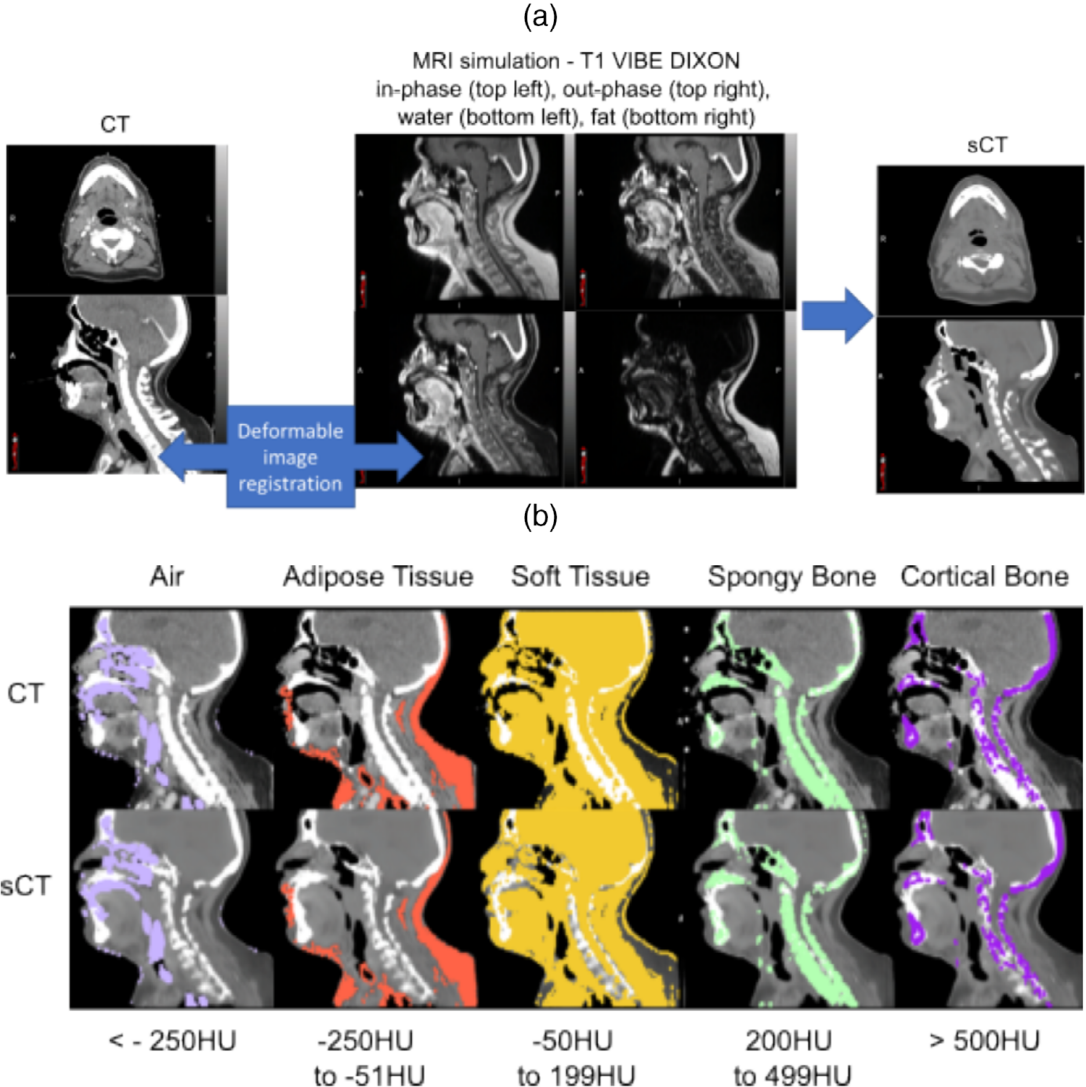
(a) Same day CT and MRI images were acquired. Deep‐learning based sCT images were generated using chain deformed T1‐VIBE Dixon MRI images. (b) Soft tissue (yellow), adipose tissue (orange), air (lilac), spongy (green), and cortical bone (purple) were segmented on CT and sCT allowing for quantification of HU differences.
